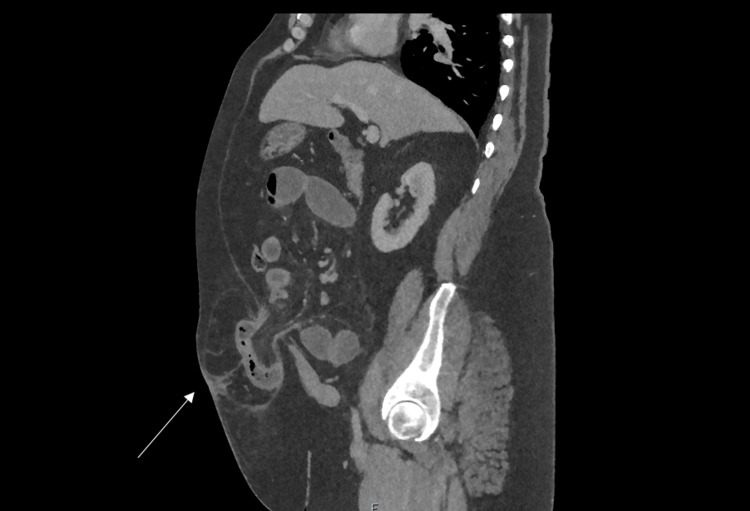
CT abdomen showing a sagittal view of a paraumbilical hernia containing bowel, causing small bowel obstruction.
